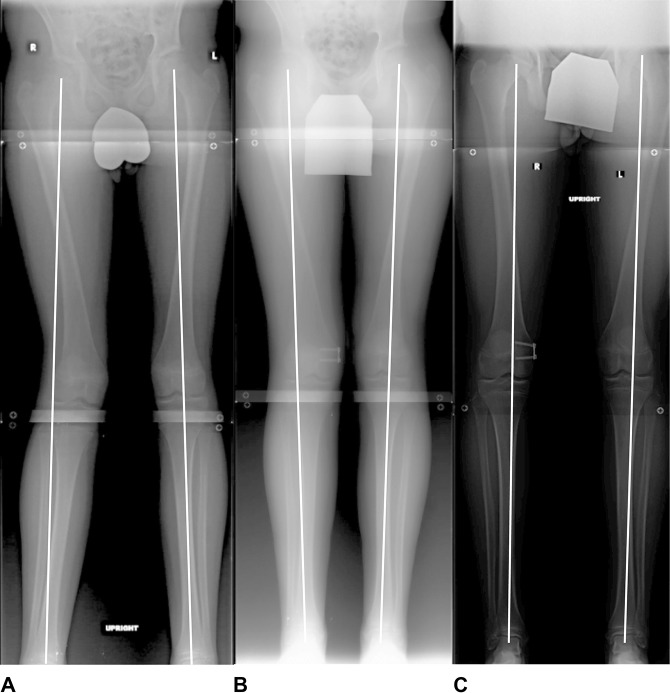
Serial standing alignment radiographs of an adolescent boy (patient #1) with lateral distal femoral hemiphyseal arrest treated with guided growth until resolution of his mechanical axis. A, demonstrates the patient’s presenting radiographs. B, represents the patient’s radiographs 4 months after starting guided growth with a medial tension band. C, represents the patient 15 months after initiation of guided growth with restoration of his mechanical axis.
